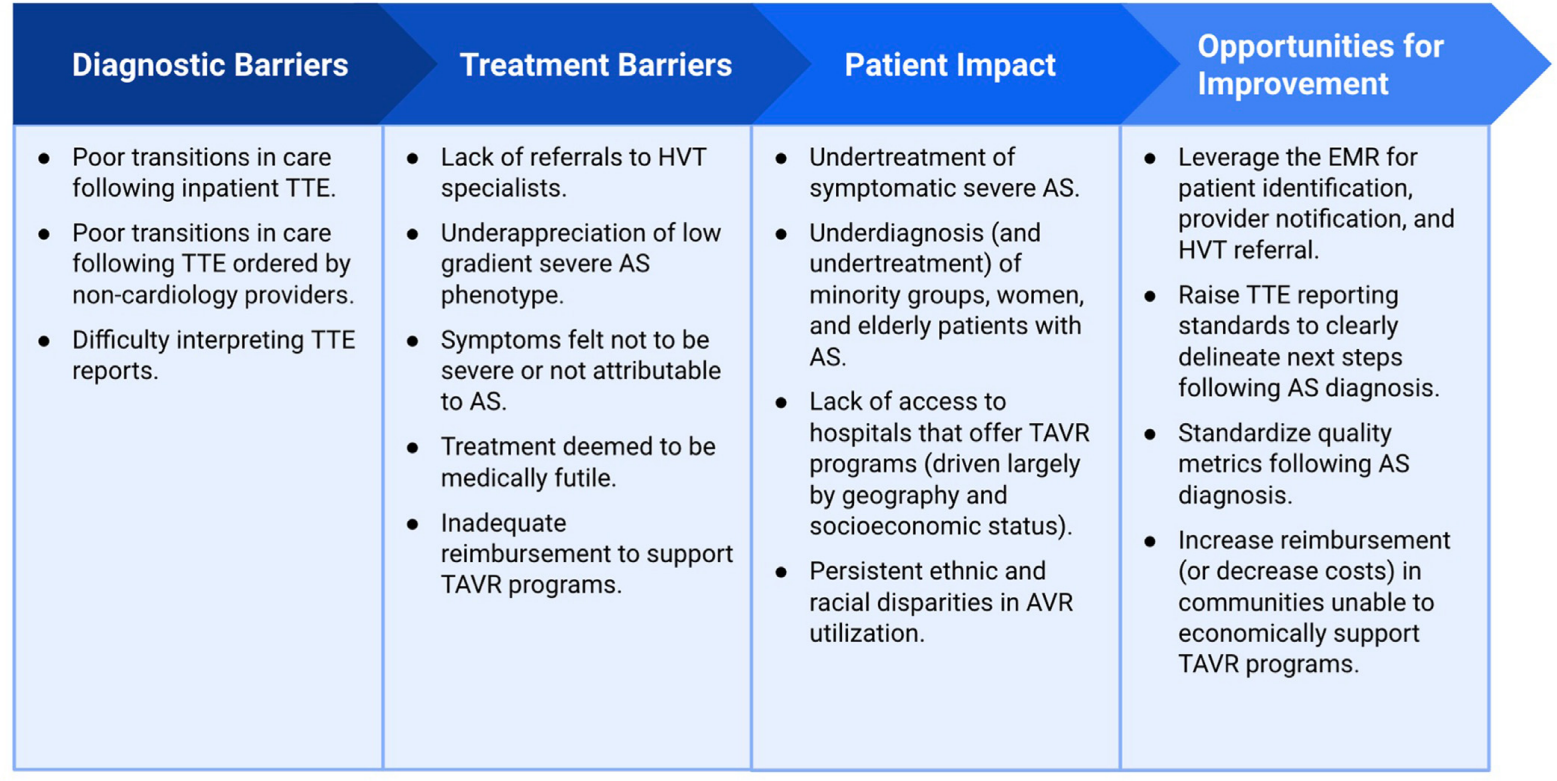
Obstacles and opportunities in the management of AS. Abbreviations: AS, aortic stenosis; AVR, aortic valve replacement; EMR, electronic medical record; HVT, heart valve team; TAVR, transcatheter aortic valve replacement; TTE, transthoracic echocardiogram.
